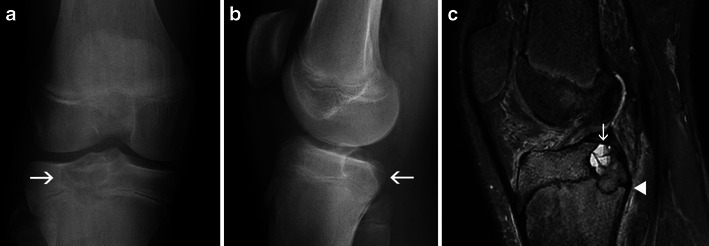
Chondroblastoma of the proximal tibial epiphysis: Frontal (a) and lateral (b) X-rays of the right knee demonstrate an osteolytic epiphyseal lesion, crossing the open physis and extending into the metaphysis. Sagittal fat-saturated proton density-weighted MRI (c) confirms the lesion and the extension across the physis (*arrowhead*). Note the presence of fluid-fluid levels (*arrow*)
